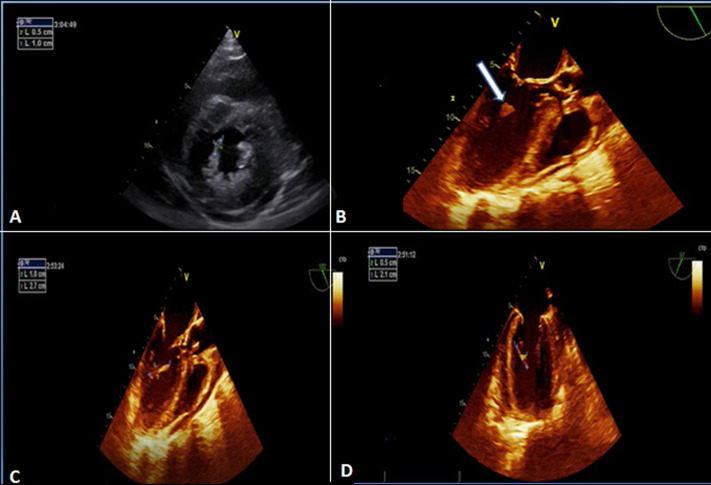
vegetation on the mitral valve on echocardiogram; transthoracic echocardiogram: vegetation on the posteromedial mitral valve pillar shown in short axis view (A); this vegetation was more studied on transoesophageal echocardiogram (B, C, D)
